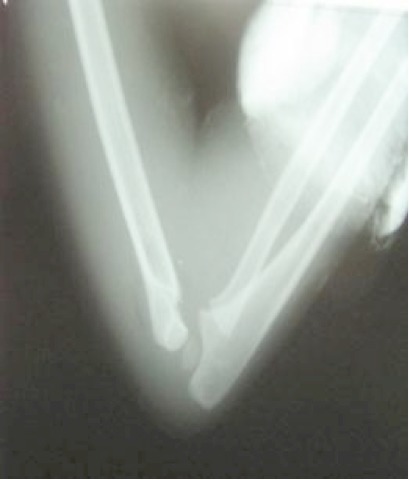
Radiographie de contrôle de profil après manœuvre de Blount montrant la bonne reduction
